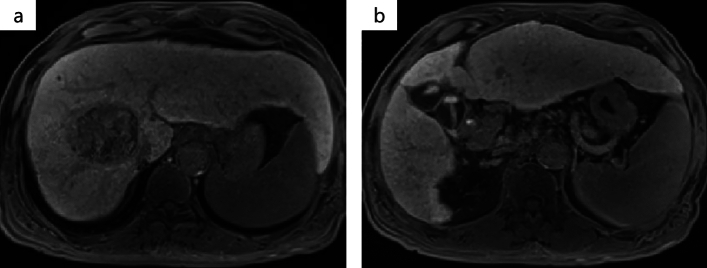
EOB-MRI after 5 courses of Atezo/bev and 3 times atezolizumab monotherapy. a In the hepatocyte phase, the main tumor continued to shrink. b In the hepatocyte phase, other lesions showed no lower signal than the surrounding normal liver tissue
